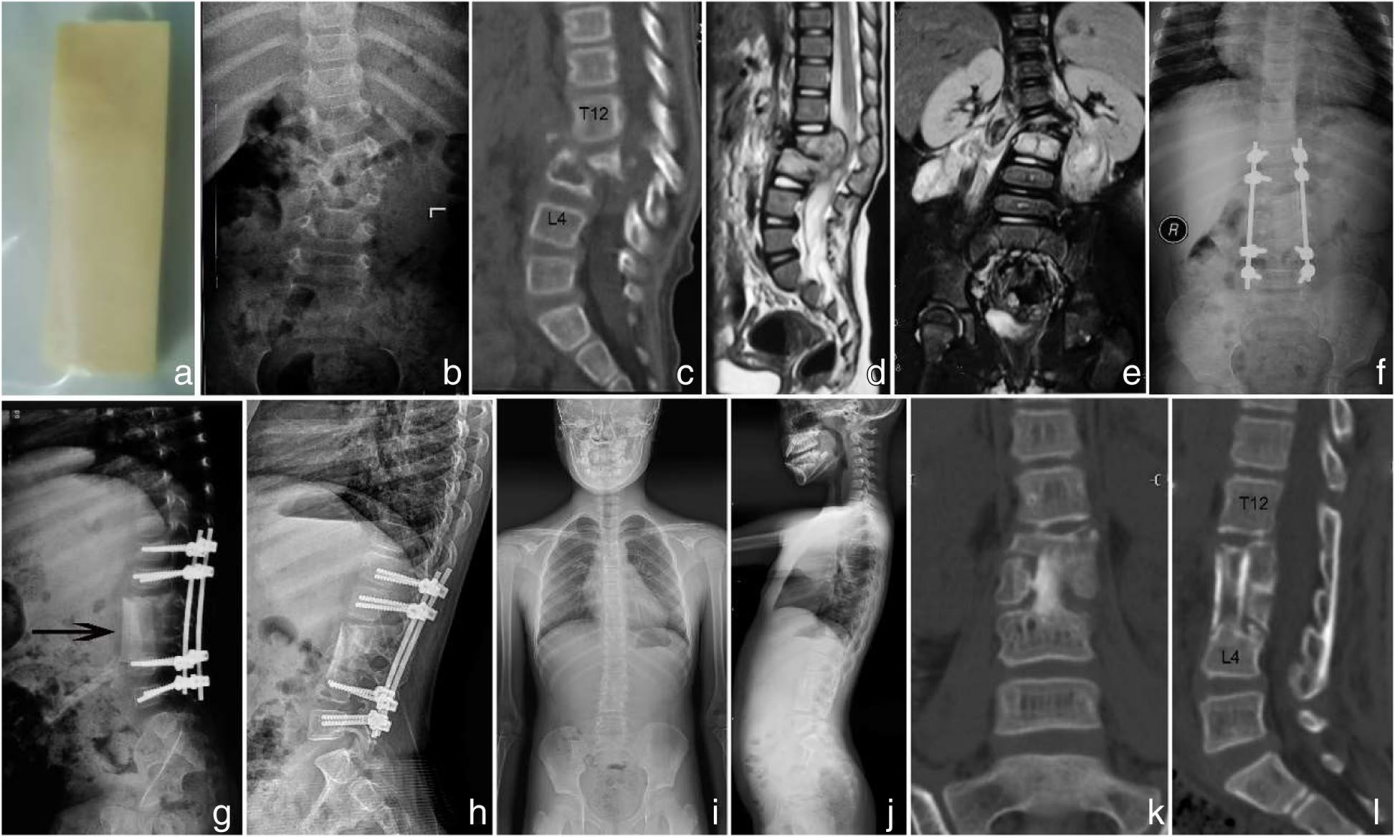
Allogenic strut bone (a). Preoperative imaging of a 3-year-old girl showing severe destruction of the vertebral body at L1-L3, with kyphosis, spinal cord compressed, and bilateral extensive abscesses (b-e). Posterior instrumentation combined with anterior debridement and reconstruction using allogenic strut bone was performed. Postoperative radiographs revealed perfect correction of kyphosis (f, g). Two-year postoperative X-ray showed a good position of the internal fixation, and the strut bone obtained reshape (h). Coronal and sagittal alignment of the patient were satisfactorily maintained 6 years postoperatively (i, j). Solid fusion was achieved (k, l)
